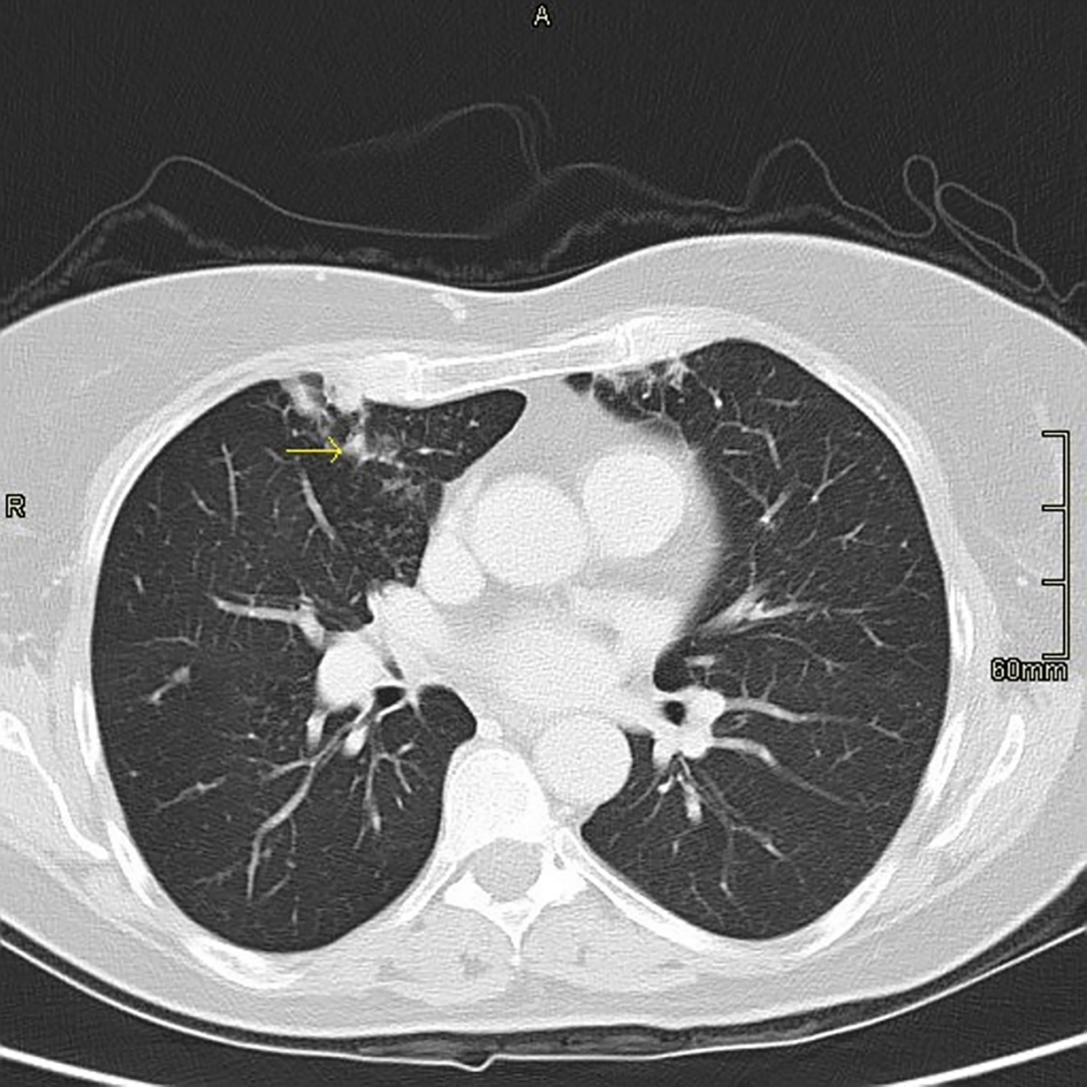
CT chest showing tree-in-bud infiltrates and diffuse nodularity.
